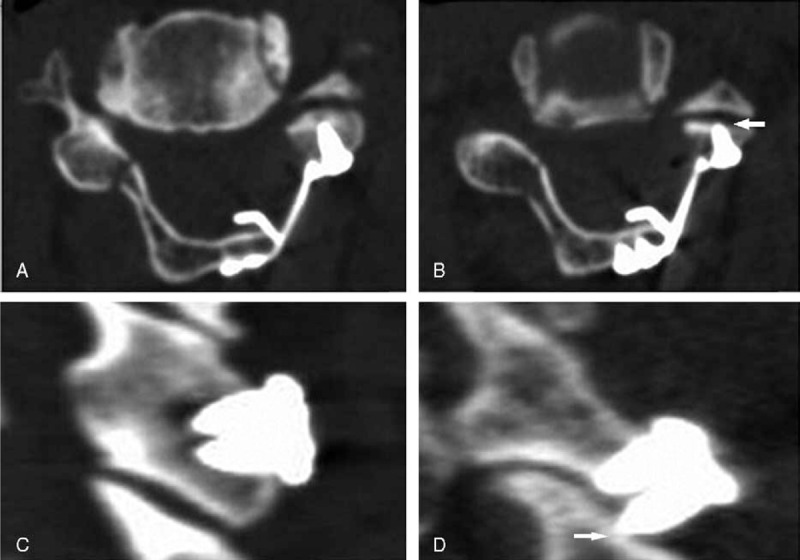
The identification of facet joints disturbance caused by miniscrews. (A, B) The axial CT images; (C, D) the sagittal CT images. (A, C) The screws did not penetrate into the facet joints; (B, D) the screws penetrated into the facet joints (the arrows).
